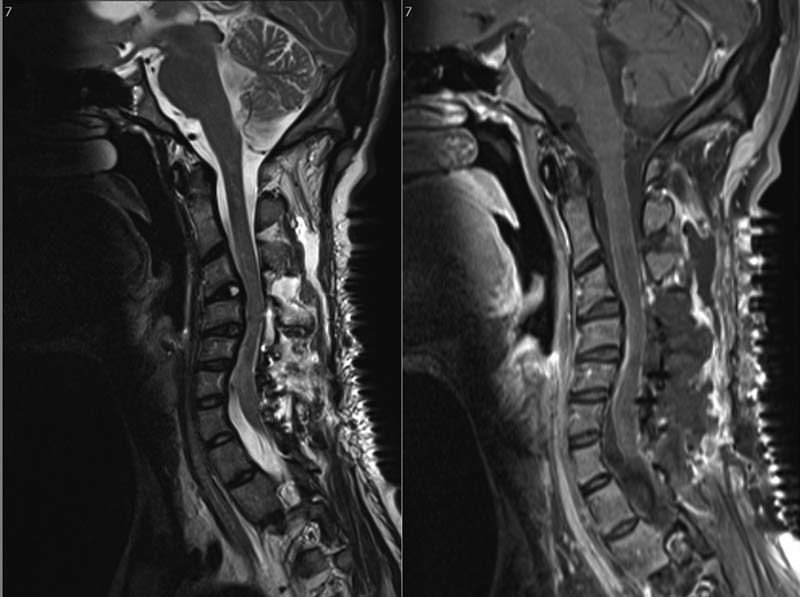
Sagittal (left) T2-weighted and (right) T1-weighted postoperative magnetic resonance imaging of the second case showing complete removal of the tumor.
